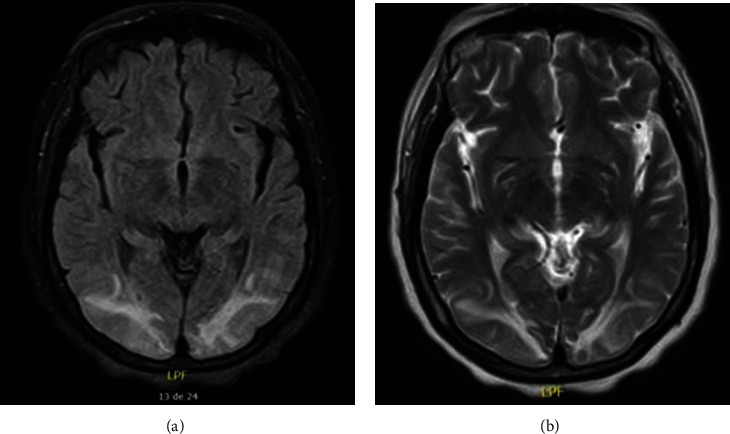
Brain MR of case 1. (a) Fluid-attenuated inversion recovery sequence showing occipital hyperintensities in occipital gyri. (b) T2-weighted sequence of the same patient showing hyperintensities in the occipital lobe.
